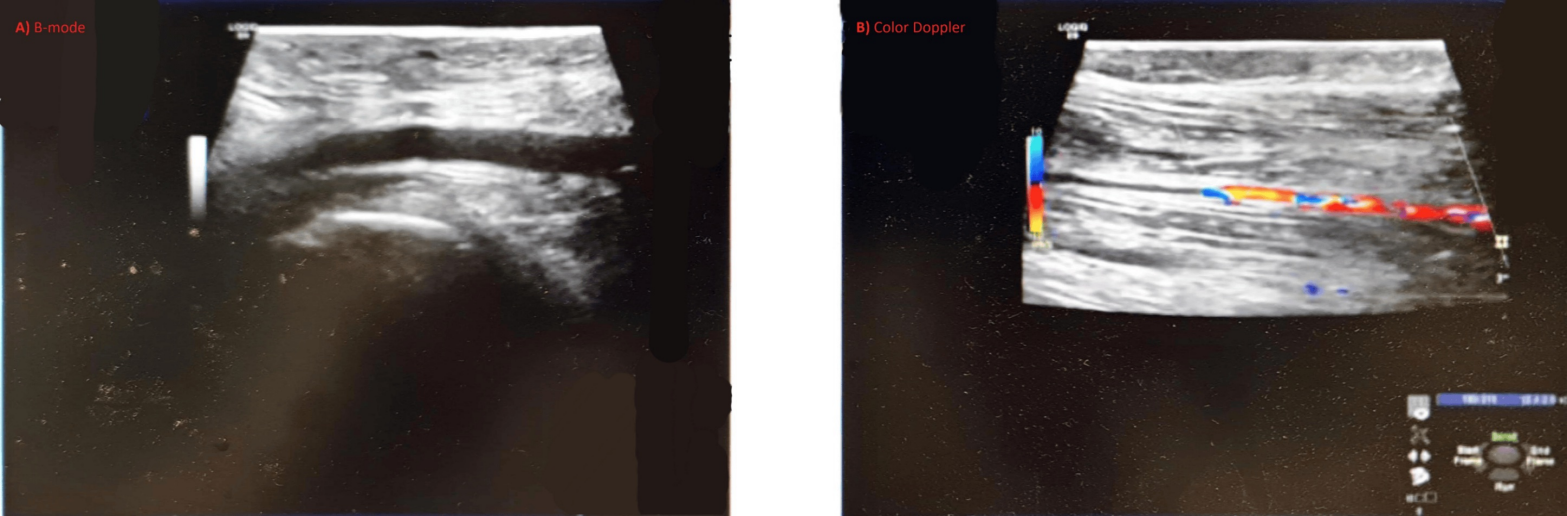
Lower-Limb Venous Doppler Ultrasound Bilateral lower-limb venous Doppler ultrasound showed patent deep veins with no evidence of thrombosis.
